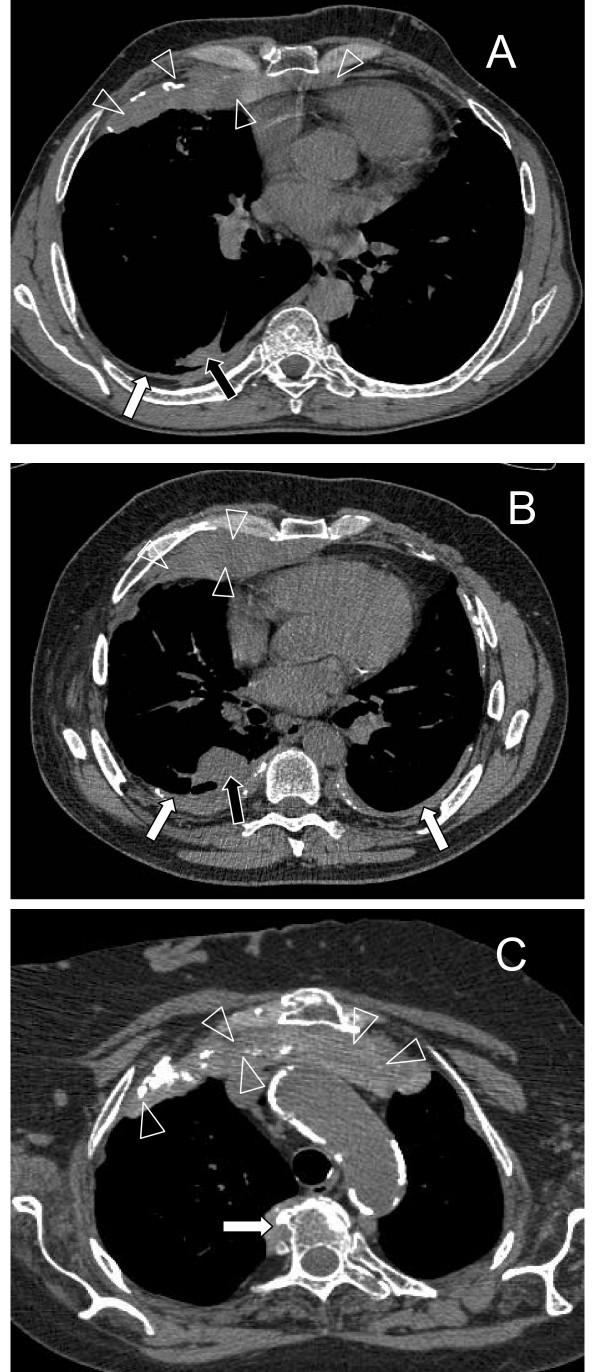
Pleural masses in the patients with retroperitoneal fibrosis (RPF). A: High-resolution computed tomography scan of the lung of a 55-year-old former pipefitter with RPF; there is a large anterior pleural mass (maximum dimensions of 30 mm × 150 mm) continuing into the mediastinum (arrowheads) and a thinner dorsal diffuse pleural thickening (white arrow) with a rounded atelectasis (black arrow); the patient had undergone left-sided pleural decortication 10 years earlier. B: An anterior mediastinal mass with maximum dimensions of 30 mm in thickness and 190 mm in width (arrowheads), bilateral diffuse pleural thickening (white arrows) with a rounded atelectasis on the right side (black arrow); this 62-year-old RPF patient had worked as a storeman, had used asbestos gloves and sealing tapes and done some pipe insulation. C: A 76-year-old female with RPF worked as a construction cleaner and had had a high level of asbestos exposure; there is a large plaque-like mass with calcifications with maximum dimensions of 27 mm in thickness and 150 in mm width. A smaller paravertebral plaque (white arrow) has no continuity with retroperitoneal fibrosis.
